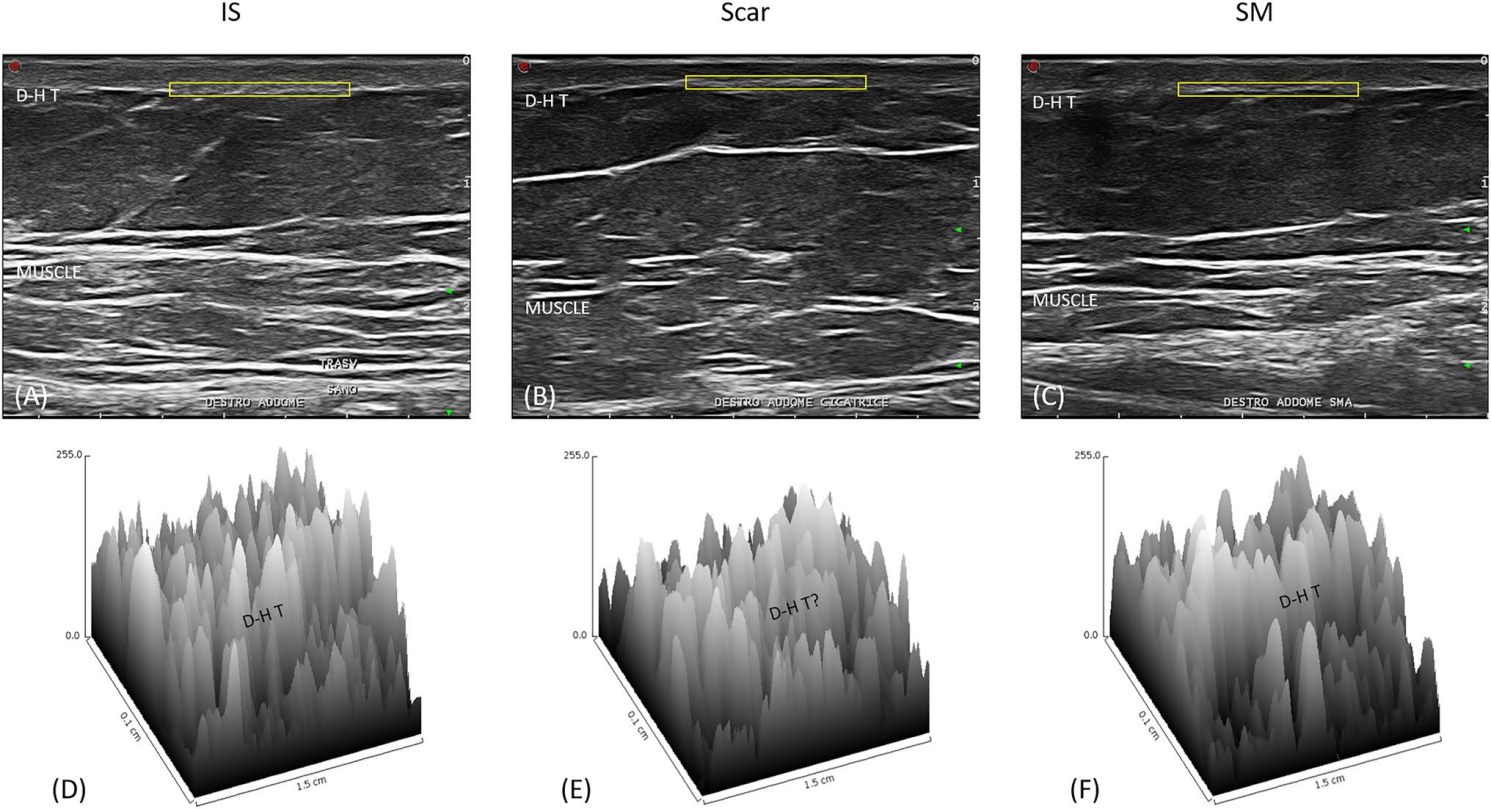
Transversal section of ultrasound images. (A) The transverse pattern of the IS is similar to the longitudinal one. (B) The transverse pattern of the scar is similar to the longitudinal one. (C) In the transversal image of the SM, the alteration of the connective tissue texture of the hypodermis is more evident than in the longitudinal section. The texture is similar to that of the scar. (D) Three-dimensional reconstruction of the dermal-hypodermal transition of the IS confirms the clear separation between the dermis and hypodermis. (E) Three-dimensional reconstruction of the dermal-hypodermal transition of the scar confirms the prominent dermal alteration. (F) The three-dimensional reconstruction of the dermal-hypodermal transition of the SM highlights an intermediate structure between that of the IS and that of the scar. D-H T = Dermal-hypodermal transition
